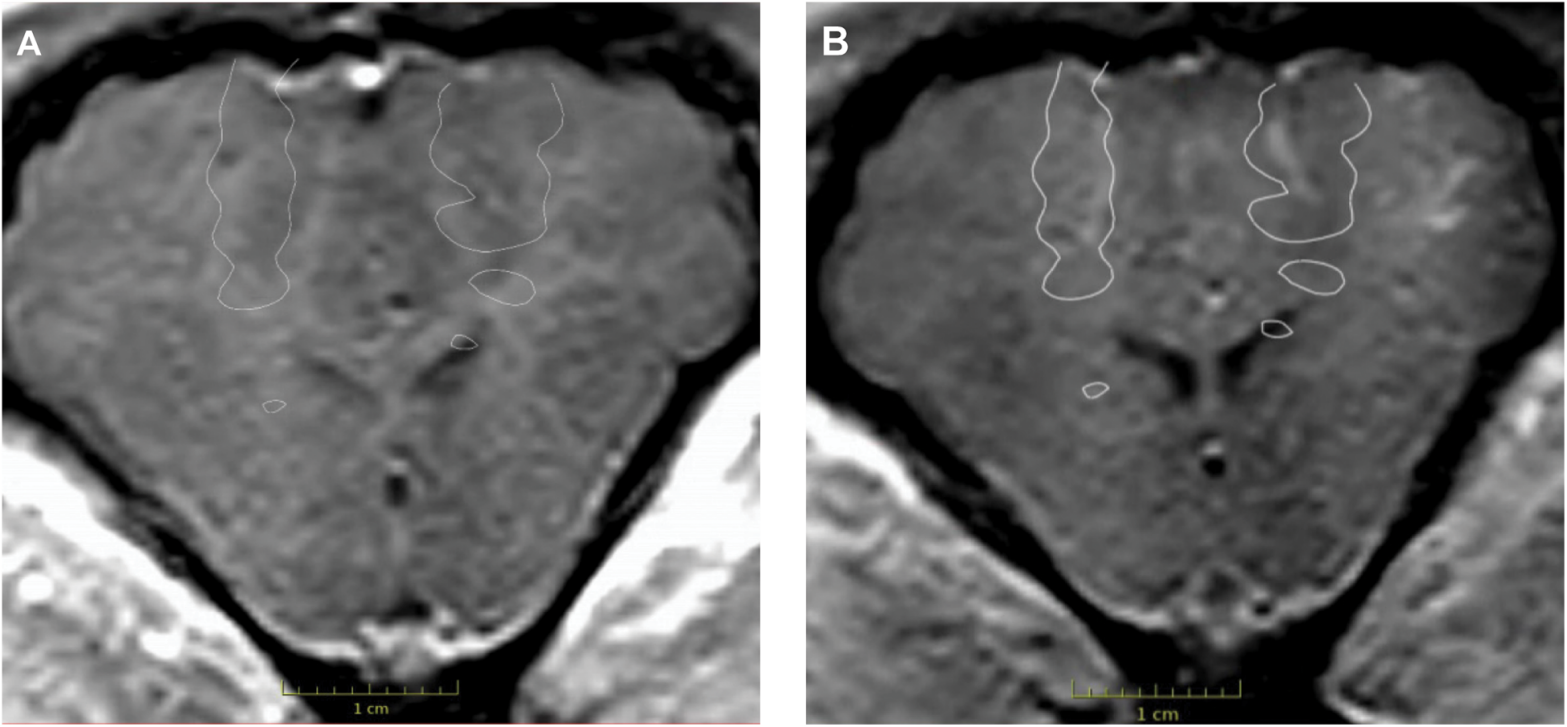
Contrast-enhanced T1W MRI images were acquired before and after the FUS procedure. Contrast enhancement was absent on the pre-procedural MRI (A) but present bilaterally on the post-procedural MRI (B) The white outlines in both images indicate the planned target for the FUS procedure. Used with permission from Barrow Neurological Institute, Phoenix, Arizona.
